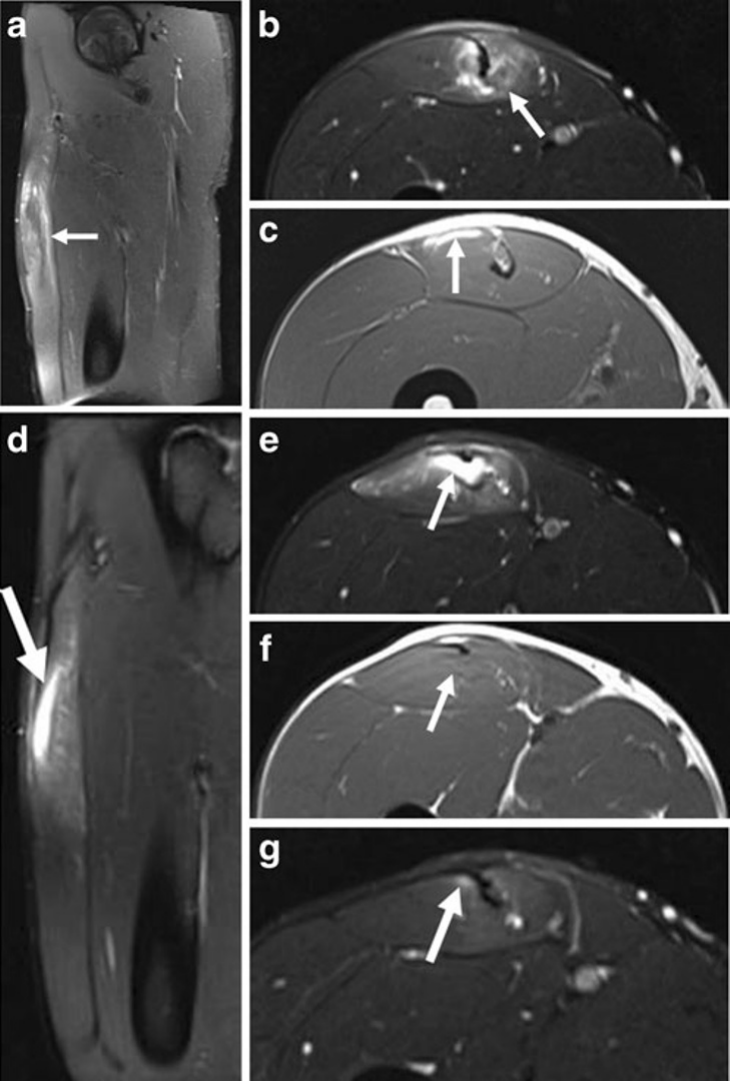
Follow-up MR images of a 20-year-old professional football player with a history of partial tear of the proximal third of the musculotendinous junction of the rectus femoris muscle 3 weeks prior. The patient presented with a re-injury after resuming competitive activity. a Sagittal and (b) axial FS T2-w images and (c) axial T1-w image. Extending distally from the proximal musculotendinous junction, a multisegmental intratendinous partial tear of the rectus femoris is demonstrated with significant peritendinous muscle oedema (*arrows*). c Epifascial intramuscular hyperintensity on T1-w image in the anterior rectus femoris represents acute haemorrhage (*arrow*). At 5-month follow-up, (d) sagittal and (e) axial FS T2-w images show focal, full-thickness tendon disruption involving the deep tendon of the rectus femoris muscle (*arrows*). There is a musculotendinous gap, filled with fluid (*arrow*), at the level of the mid-thigh measuring 3.4 cm in length. The fluid collection represents liquefied residual haematoma with hyperintensity on the (e, *arrow*) FS T2-w TSE image and isointensity to hypointensity on the (f, *arrow*) T1-w image. Some of the fibres of the intramuscular tendon remain intact. g Axial FS T2-w image at 6.5-month follow-up demonstrates only very discrete peritendinous oedema adjacent to the tendon (*arrow*)
